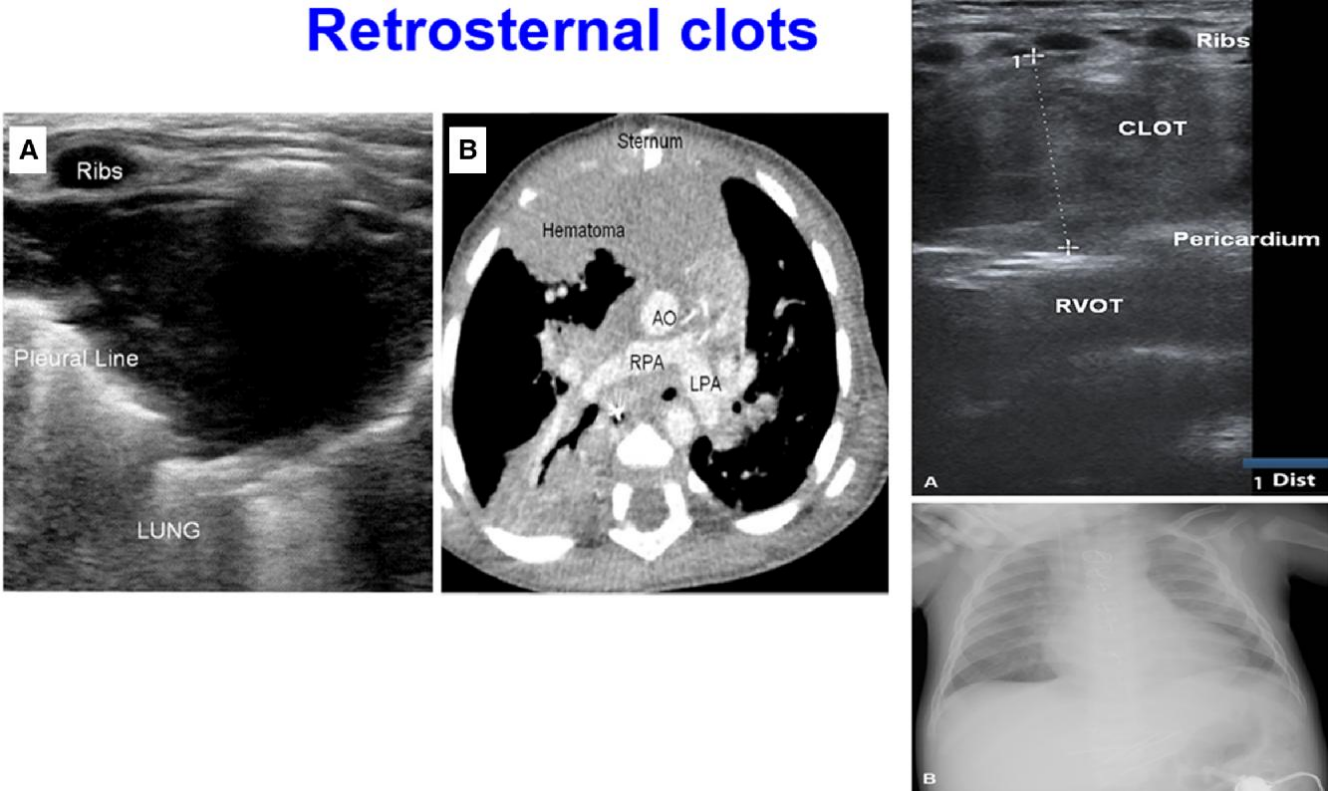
Retrosternal clot. (Left) In (*A*), a retrosternal clot is visualized by LUS and confirmed (*B*) by CT scan. Using LUS, it is possible to appreciate how the clot is interposed among the sternum and the pleural line. (Right) A retrosternal clot among the sternum and the right ventricular outflow tract (RVOT) is visualized by LUS (*A*). On chest X-ray, enlargement of the right mediastinum can be observed.
